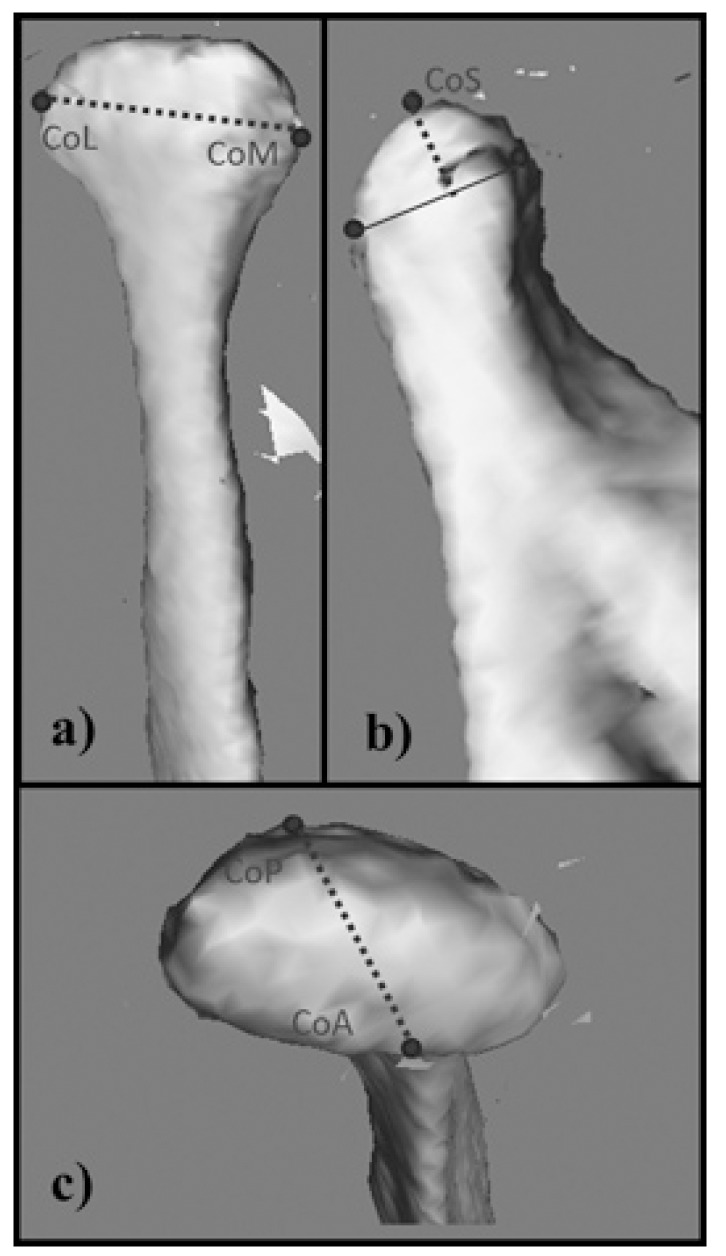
Condyle measurements performed on the 3D-CT model.
